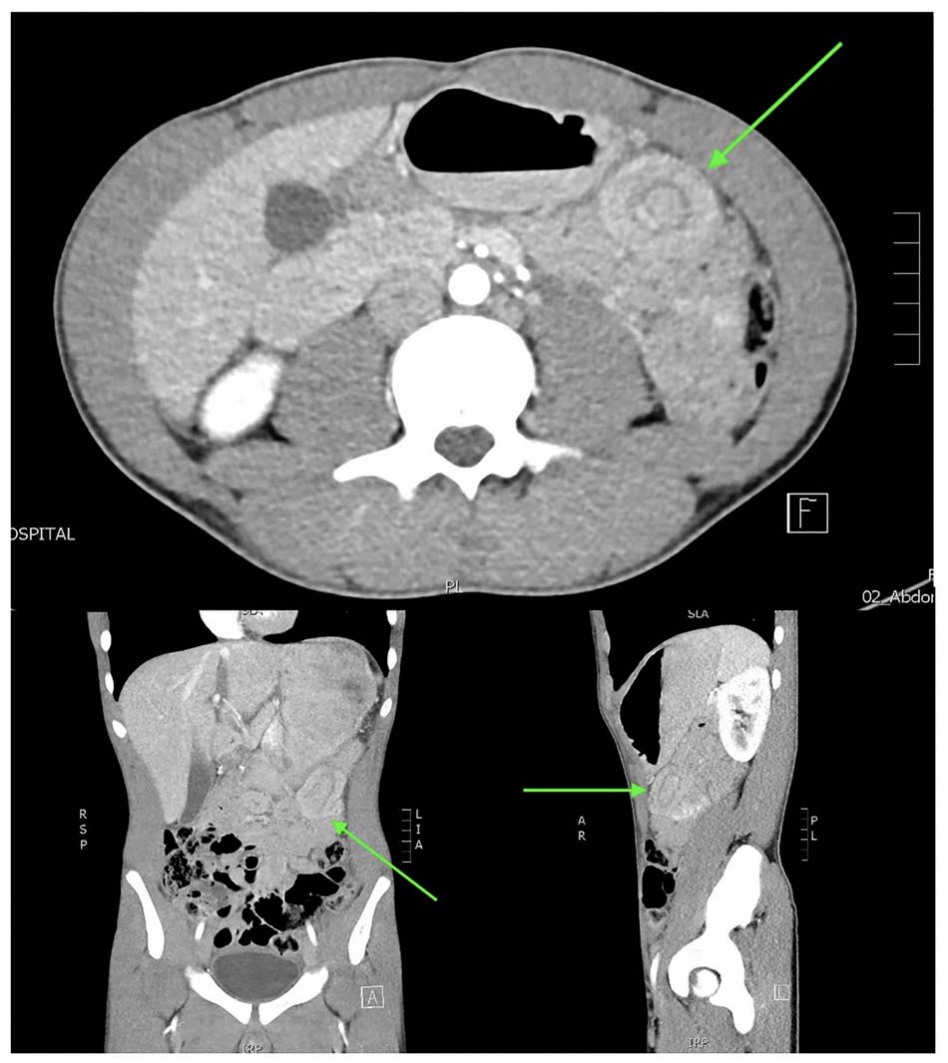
Abdominal CT in axial, coronal and sagittal planes demonstrating mesenteric haematoma and jejuno-jejunal intussusception (‘bowel-within-bowel’ sign indicated by green arrow).
